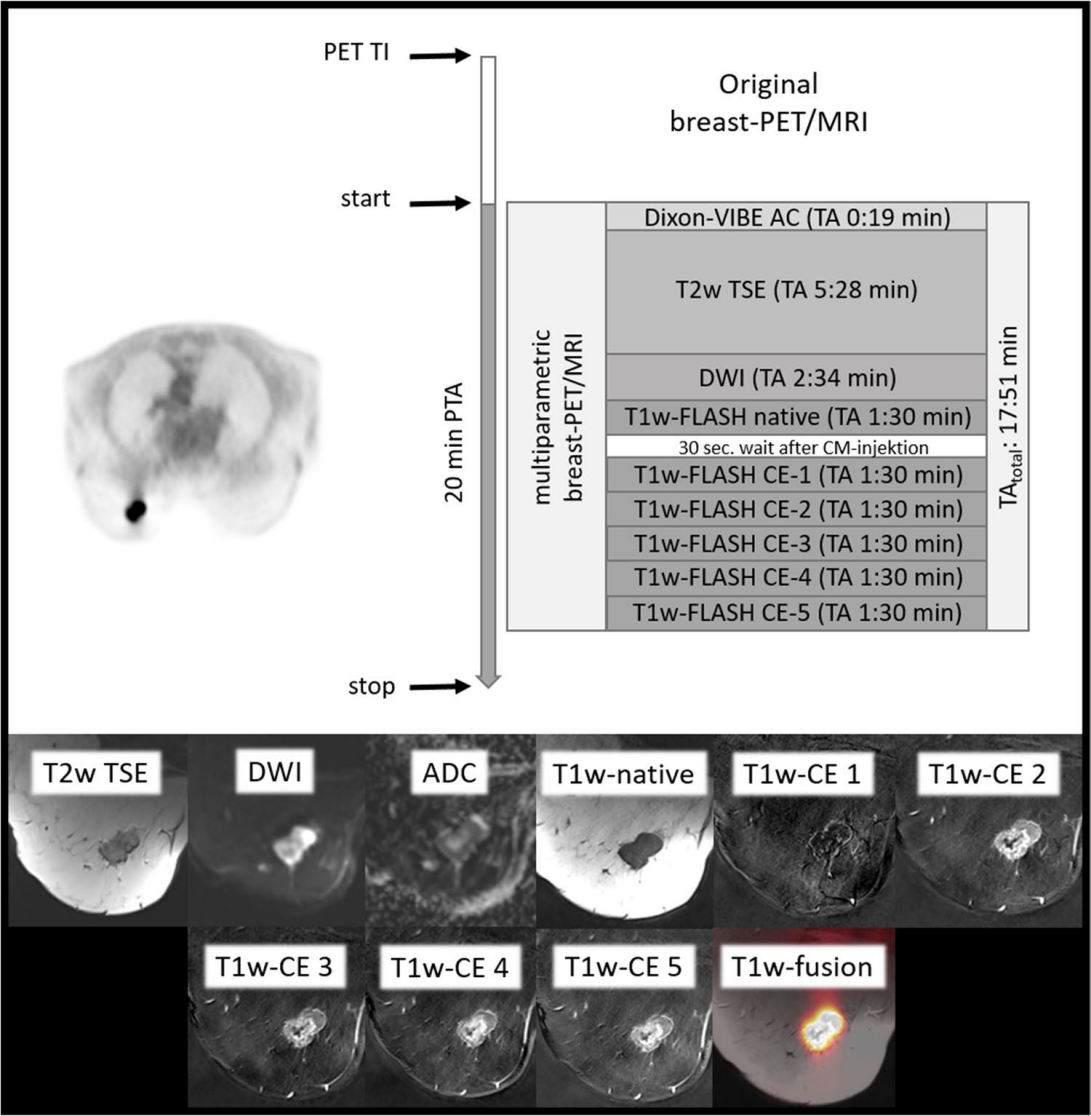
Overview of the original comprehensive breast PET/MRI protocol after tracer injection (TI) and acquisition time (TA) of each MR sequence and simultaneous PET data acquisition with a PET acquisition time (PTA) of 20 min
